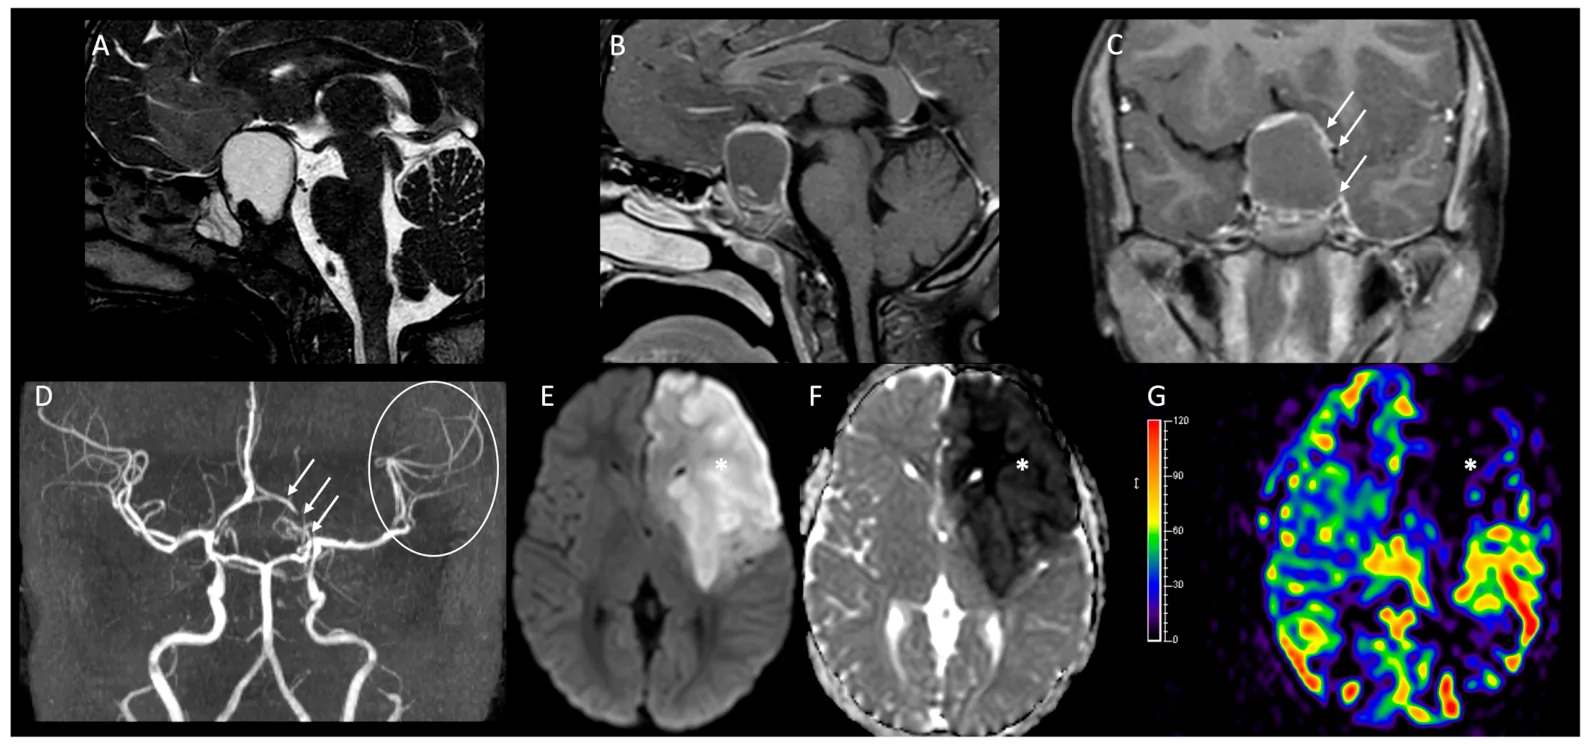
ACP. MRI/MRA study of a 6-year-old girl with right hemiparesis and vomiting. (A) Sagittal bSSFP, (B) sagittal and (C) coronal contrast-enhanced 3D T1, (D) coronal MIP (maximum intensity projection) reconstruction of 3D TOF (time-of-flight) MRA, (E) axial DWI, (F) axial ADC map, and (G) axial color-coded map of the PCASL (pseudocontinuous arterial spin labeling). Intra- and suprasellar lobulated solid/cystic lesions characterized by inhomogeneous content and peripheral enhancement (A–C). The lesion compresses the supraclinoid segment of the left internal carotid artery and its bifurcation with a reduced flow signal of the A1 segment of the left anterior cerebral artery (ACA) (C,D, arrows) and the M2–M3 segments of the left middle cerebral artery (MCA) (D, oval). Note the large area of cytotoxic edema on DWI/ADC (E,F) and reduced perfusion in the PCASL (G) involving the left fronto-insular and basal ganglia regions, which is consistent with ischemia of the left ACA and MCA territories (E–G, asterisks).
